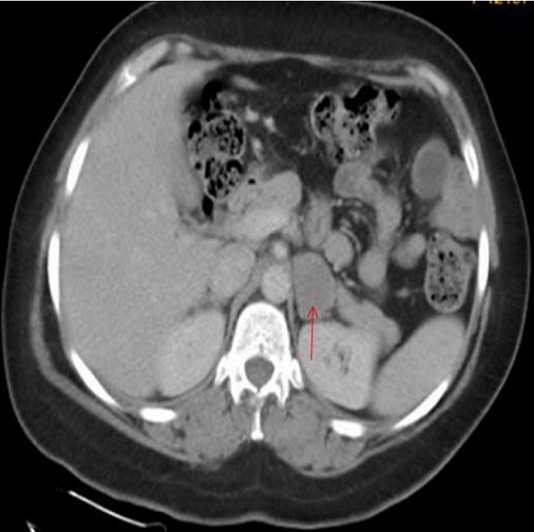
CT showed a hypodense left adrenal lesion measuring 2.5 cm X 3.4 cm in diameter with a spontaneous density of 20UH
